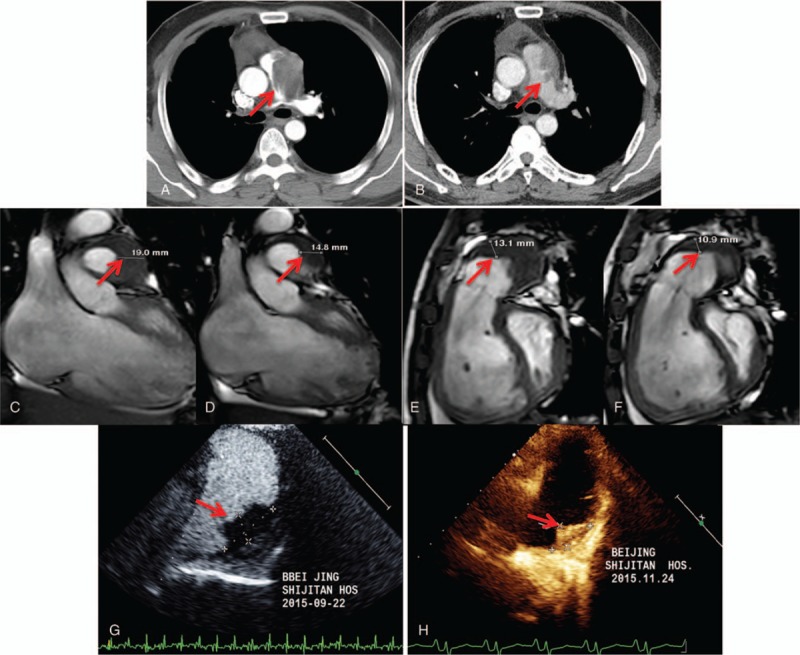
Imaging changes of before and after treatment. During 31 months follow-up, pulmonary CT demonstrated the lesion of pulmonary artery IgG4-RD in patient 1 shrunk obviously (A: before treatment, B: after treatment). During 3 months follow-up, MRI (C and E: before treatment, D and F: after treatment) and ultrasonic cardiogram (G: before treatment, H: after treatment) showed the lesion of pulmonary artery IgG4-RD also shrunk obviously in patient 3. CT = computed tomography; IgG4-RD = IgG4-related disease; MRI = magnetic resonance imaging.
